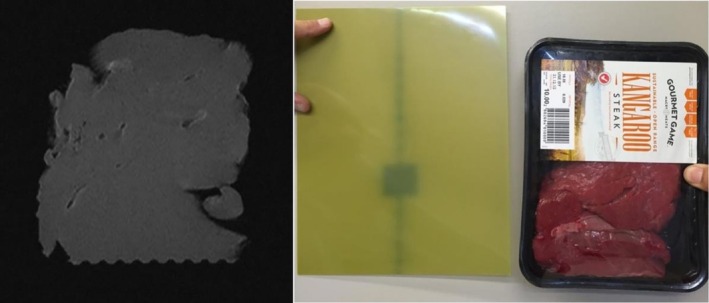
Kangaroo steak (Right) used for the first inline MRI treatment imaging (Left) [18] (with permission).
